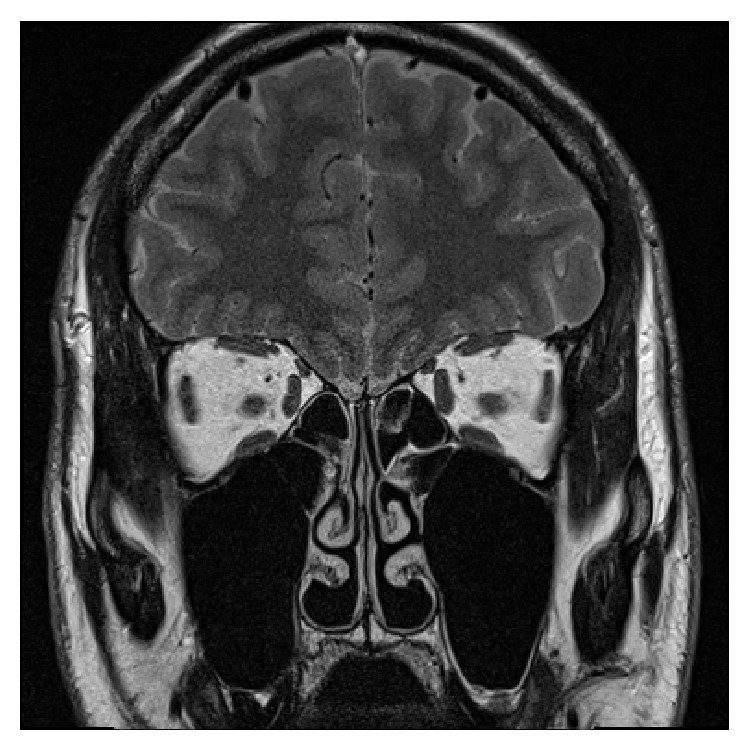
Magnetic resonance imaging showed normal sinus and brain structures.
